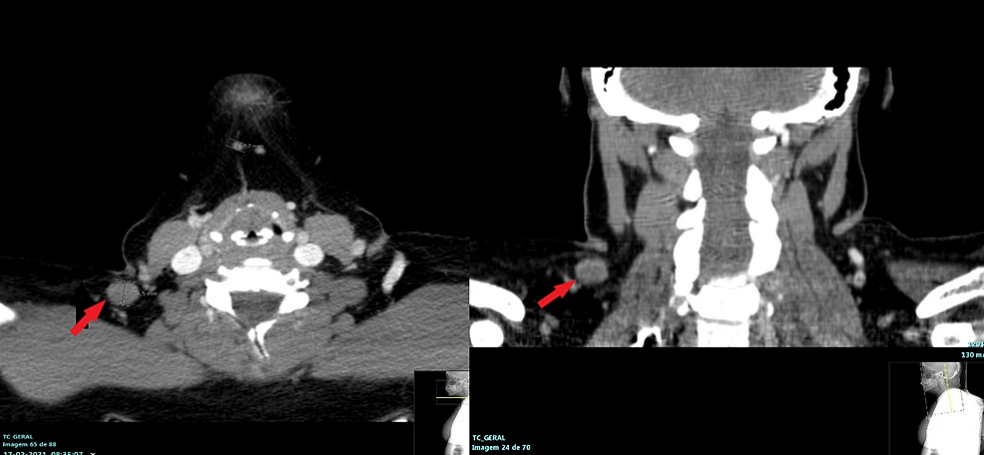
Neck CT scan - February 17, 2021 The images show a cervical ganglion of 13.7 × 13.5 mm and two adjacent others with 5 and 6 mm in size (red arrow) CT: computed tomography
